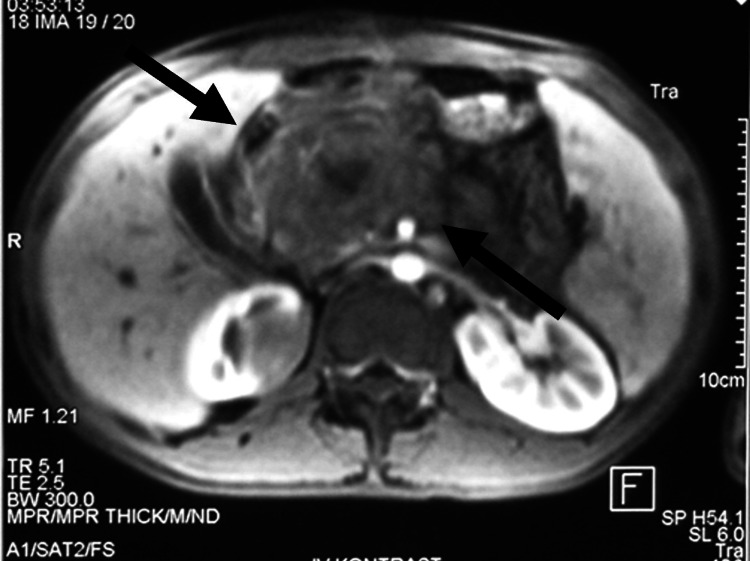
Contrast-enhanced abdominal magnetic resonance imaging showing a 5.6 × 4.2 cm heterogeneous mass (arrow) in the head of the pancreas with associated biliary dilatation and regional lymphadenopathy. A smaller lesion is visible near the upper pole of the right kidney.
